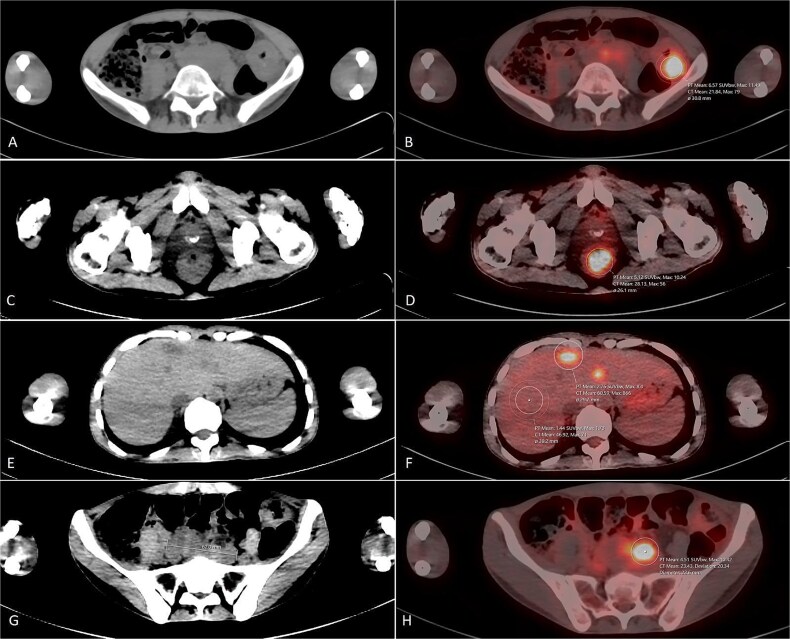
F-18 FDG PET/CT scan for the patient. Selected CT axial cuts with the corresponding PET/CT fusion images. An evidence of intensely hyper-metabolic left colonic mass is noted (A & B), in addition to another concentric intensely hyper-metabolic rectal mass just above the anal verge (C & D). Few hypo-attenuating lesions with high grade uptake were seen in the liver (E & F) as well as multiple enlarged intensely hyper-metabolic retro-peritoneal lymph nodes (G & H).
